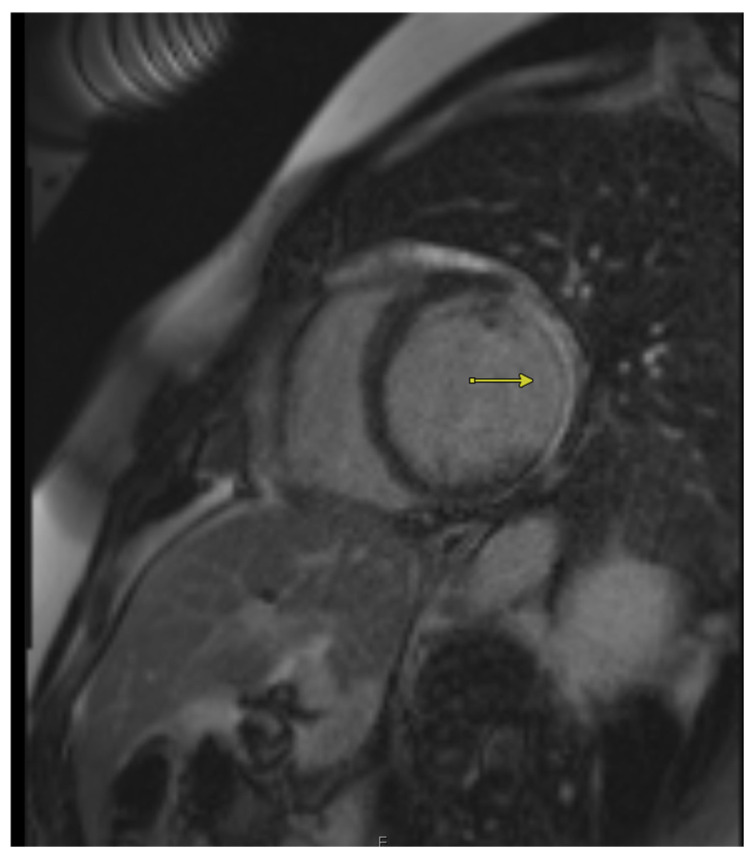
Cardiac MRI showing late gadolinium enhancement in a patient with chronic ischemic cardiomyopathy. Areas of enhancement (arrows) indicate non-viable myocardium.
